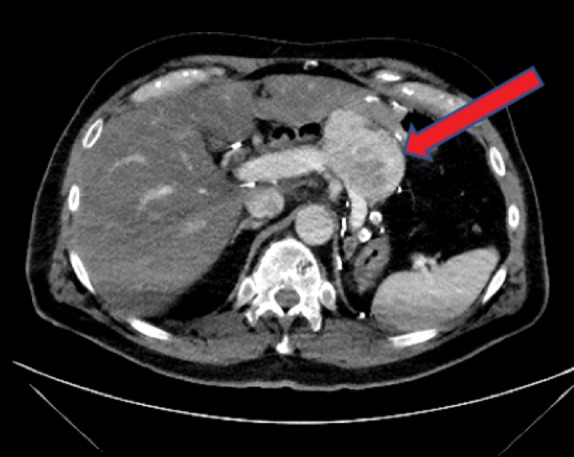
Abdominal CT scan revealing a 7 cm hypervascular mass (arrow) involving the splenic vein. Typically, RCC metastases are hypervascular with contrast enhancement, multicentric, and without peripancreatic lymphadenopathy.^2^ CT, computed tomography; RCC, renal cell carcinoma.
